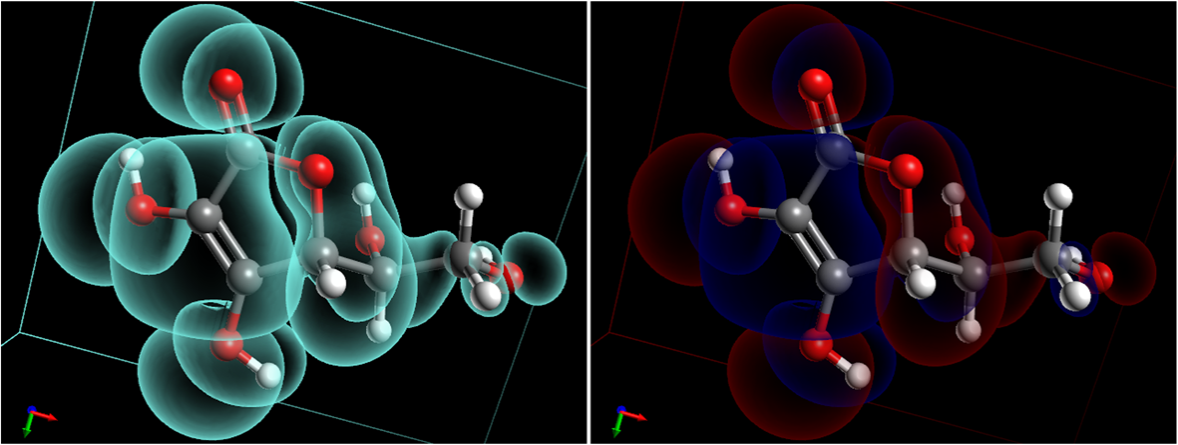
Molecular orbitals rendering using GLSL shaders. Rendering of a molecular orbital isosurface using two GLSL shaders to highlight the edges of the surfaces. The X-ray effect (left) and red and blue (right) showing the positive and negative molecular orbital shapes.
